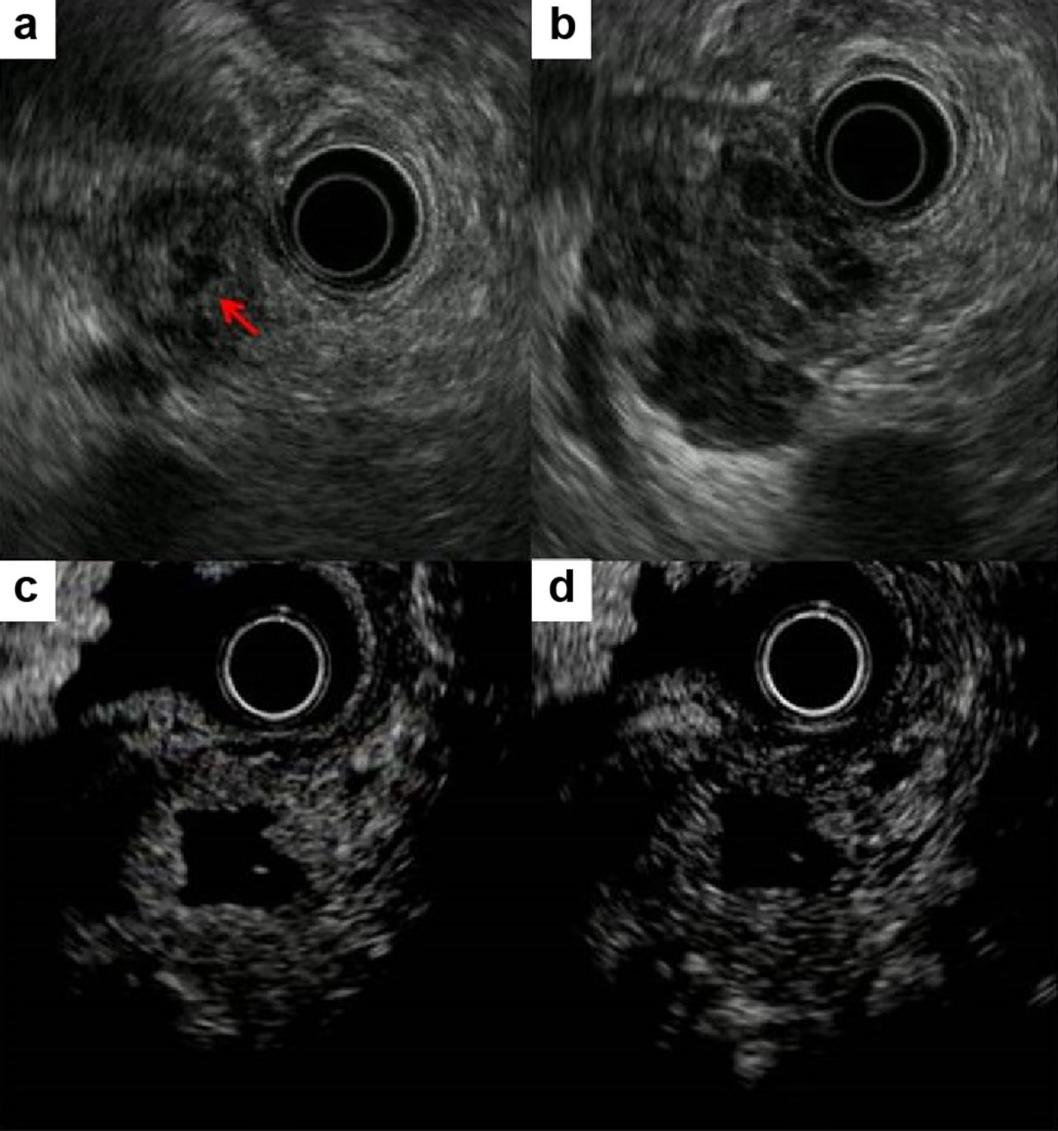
Endoscopic ultrasonography revealed hypoechoic and homogenous heterogeneous mass (a) with central anechoic areas (b, arrow). Contrast-enhanced endoscopic ultrasonography shows isoenhancement at 20 s (c) and hypoenhancement at 120 s (d) with central non-enhancement of the peripheral rim of the mass
